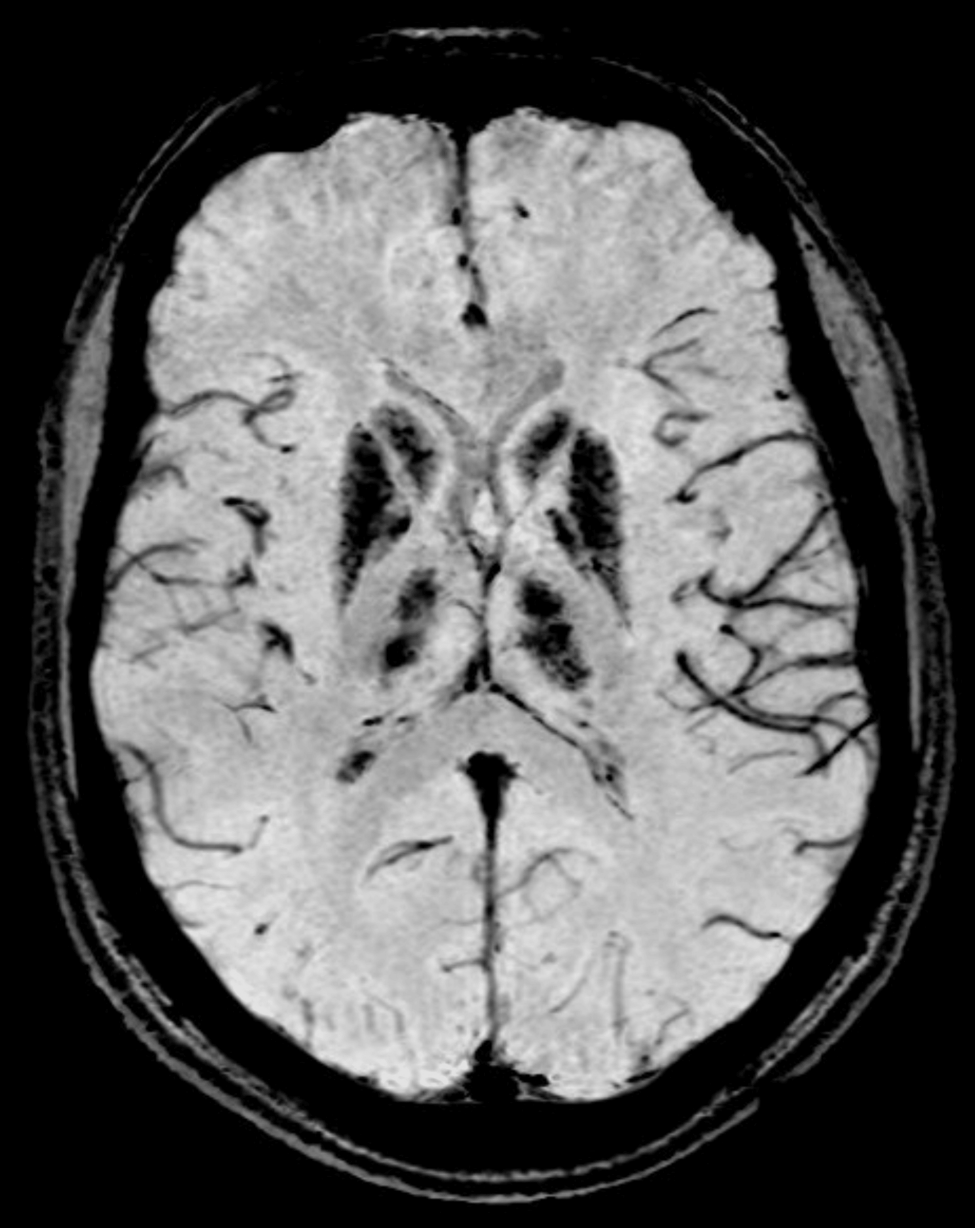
SWI (susceptibility-weighted imaging) axial MRI of the brain (plain study) image at the level of basal ganglia showing blooming in the bilateral basal ganglia, with both thalami signifying calcification
